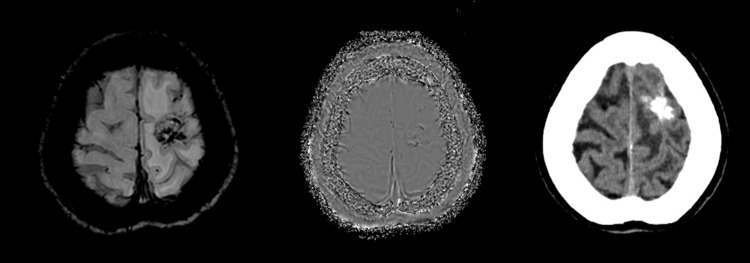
A case of lung cancer with brain metastasis where SWI shows intratumoral microbleed but appears as intratumoral calcification on brain CT This can be due to mixed microbleed and calcified components within brain metastasis CT: computed tomography; SWI: susceptibility-weighted imaging
